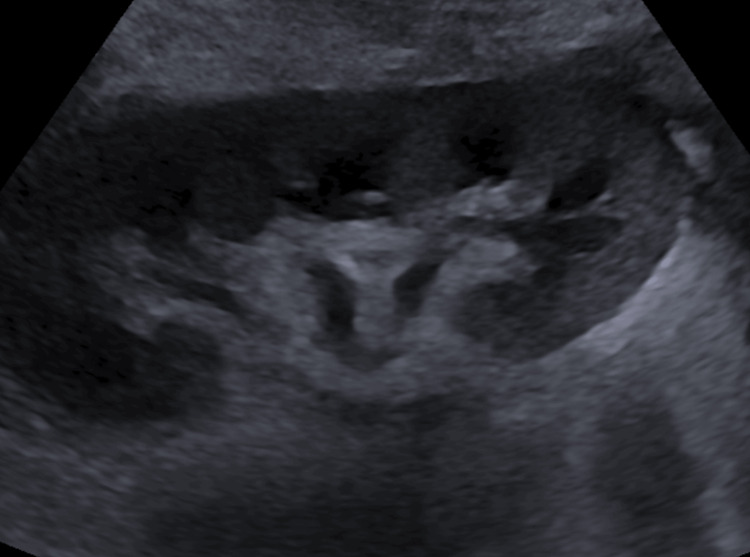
Transplant kidney ultrasound showing no evidence of hydronephrosis
